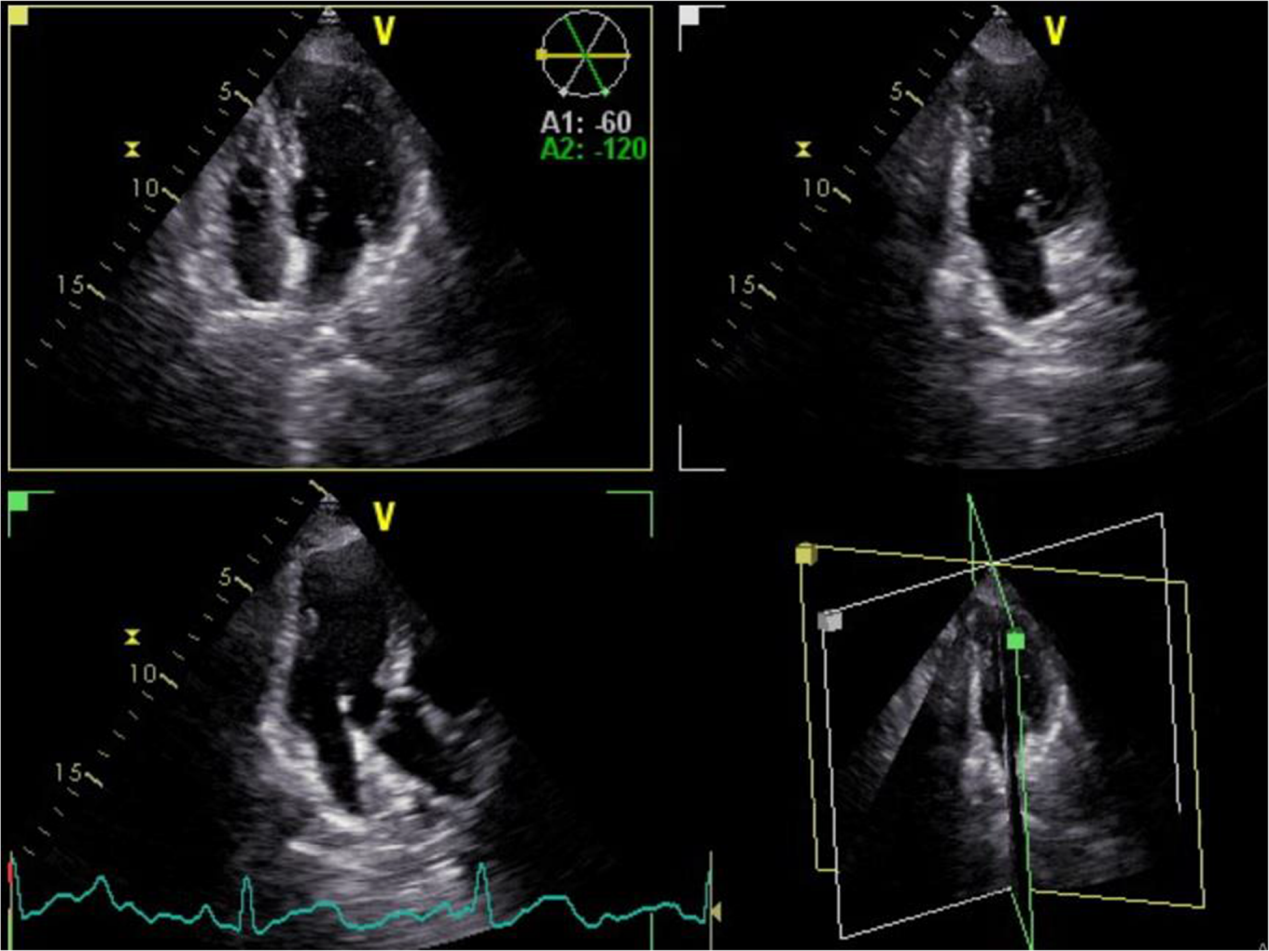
Three standard views, two-, three-, and apical four-chamber, for evaluation of longitudinal strain
